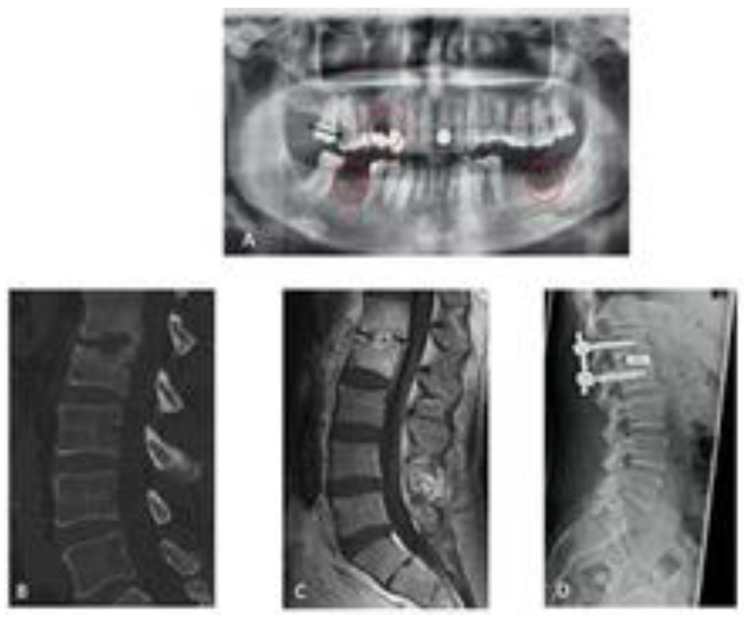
Spondylodisciits and oral cavitiy infection ((A) OPG with an infection of the oral cavity/red circels), (B,C) CT/MRT with bony destruction, (D) CT after surgical treatment of discitis).
